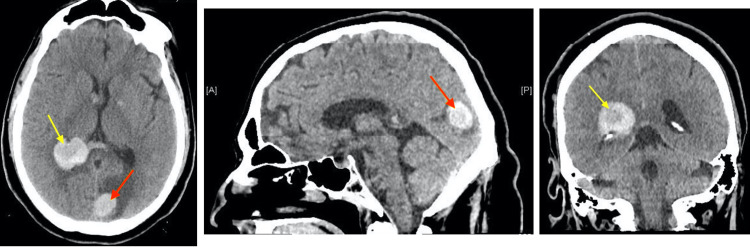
Axial, sagittal, and coronal computed tomography (CT) views demonstrating a 2 cm hyperdense lesion in the medial aspect of the left occipital lobe with moderate surrounding vasogenic edema (red arrow) and right lateral ventricular hemorrhage extending into the occipital and temporal horns (yellow arrow).
